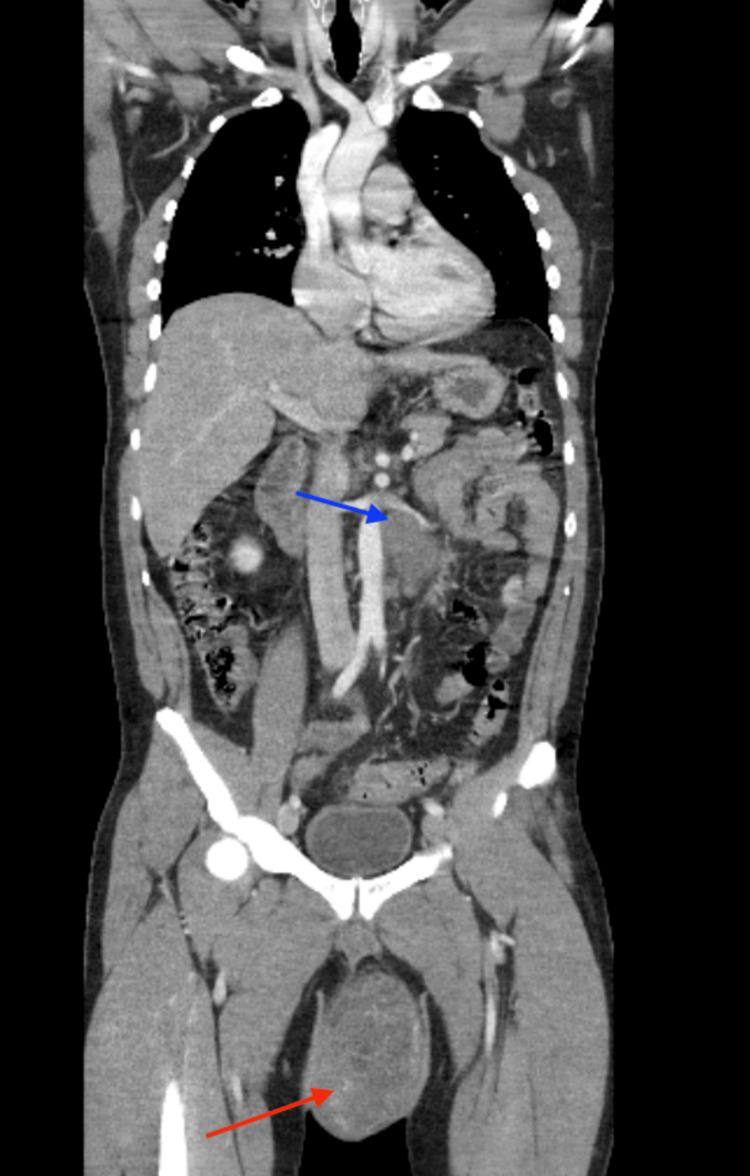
Computed tomography (CT) of the chest, abdomen, and pelvis showing a large scrotal mass (red arrow) with para-aortic lymph node metastases (blue arrow).
